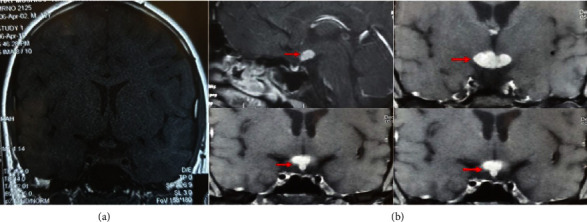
MRI sella showing (a) enhancing lesion involving hypothalamus and infundibular stalk 14 × 8 mm, isointense on T1W and hypointense on T2W at 3 years after 1^st^ presentation, and (b) increase in size of enhancing mass lesion in the upper part of stalk 20 × 17 × 15 mm, 2 years after biopsy.
